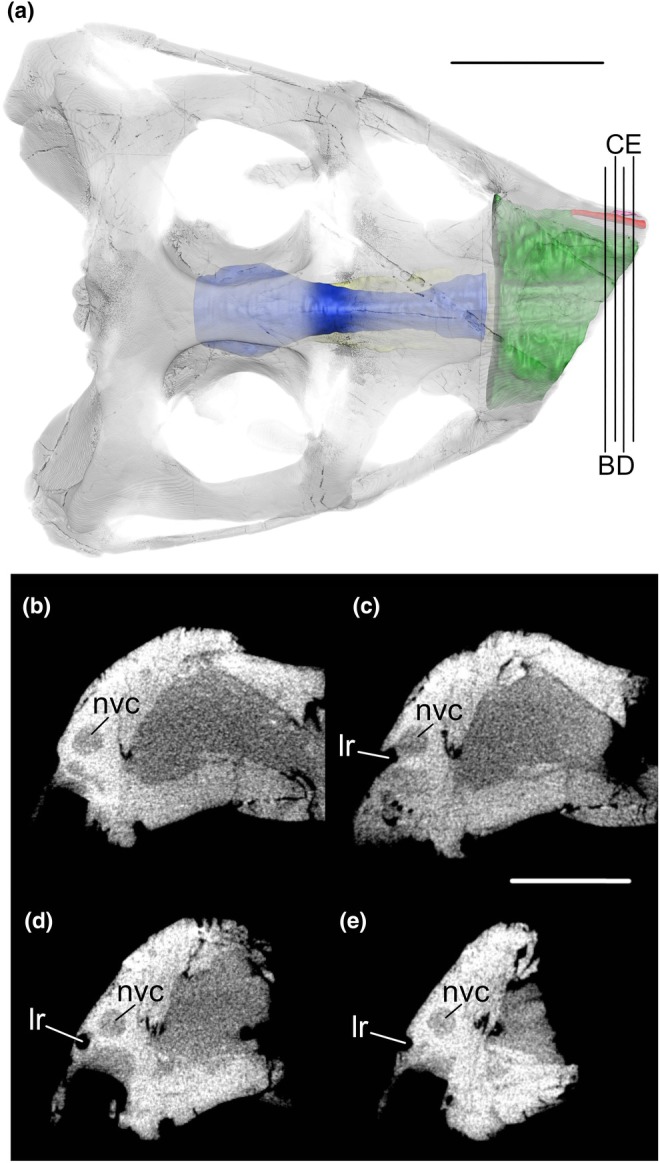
Endocast reconstruction of referred skull of *Pholidosaurus purbeckensis* (NHMUK R36721). (a) Skull in dorsal view, showing the position of the coronal CT slices. (b–e) CT slices of the olfactory region with detail of the neurovascular canal and its lateral ramification. lr, lateral ramification of the neurovascular canal; nvc, neurovascular canal. Scale bars = 50 mm (a) and 10 mm (b–e).
